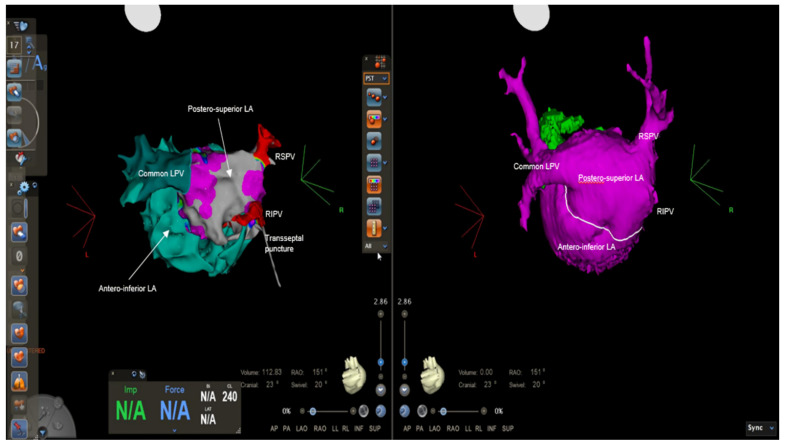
Electroanatomical mapping using CARTO 3 system and CT reconstruction showing the anatomy of the pulmonary veins: two separate pulmonary veins on the right and a common left pulmonary vein draining into the posterosuperior left-atrial chamber, where we can also notice the transseptal puncture. LA = left atrium, LPV = left pulmonary vein, RIPV = right inferior pulmonary vein, RSPV = right superior pulmonary vein.
